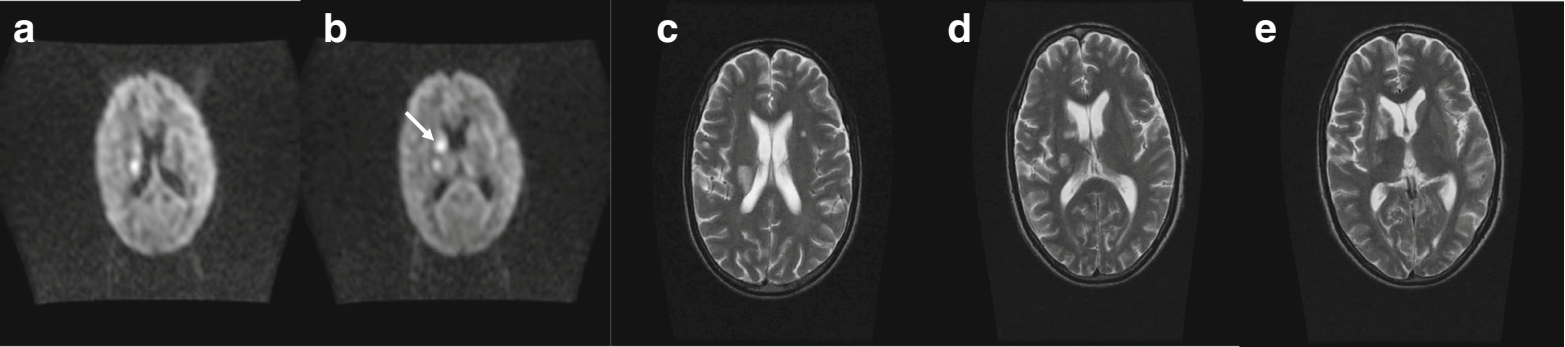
a-e are MRI sequences taken 1 month later from symptom onset and on the background of a resolved left sided weakness. a-b; shows a new DWI lesion (b – arrow) suggesting evolving inflammation. However, the burden of the previous DWI changes reported in (1) is less. c-d (T2 axial) shows maturation of previously reported infarct
